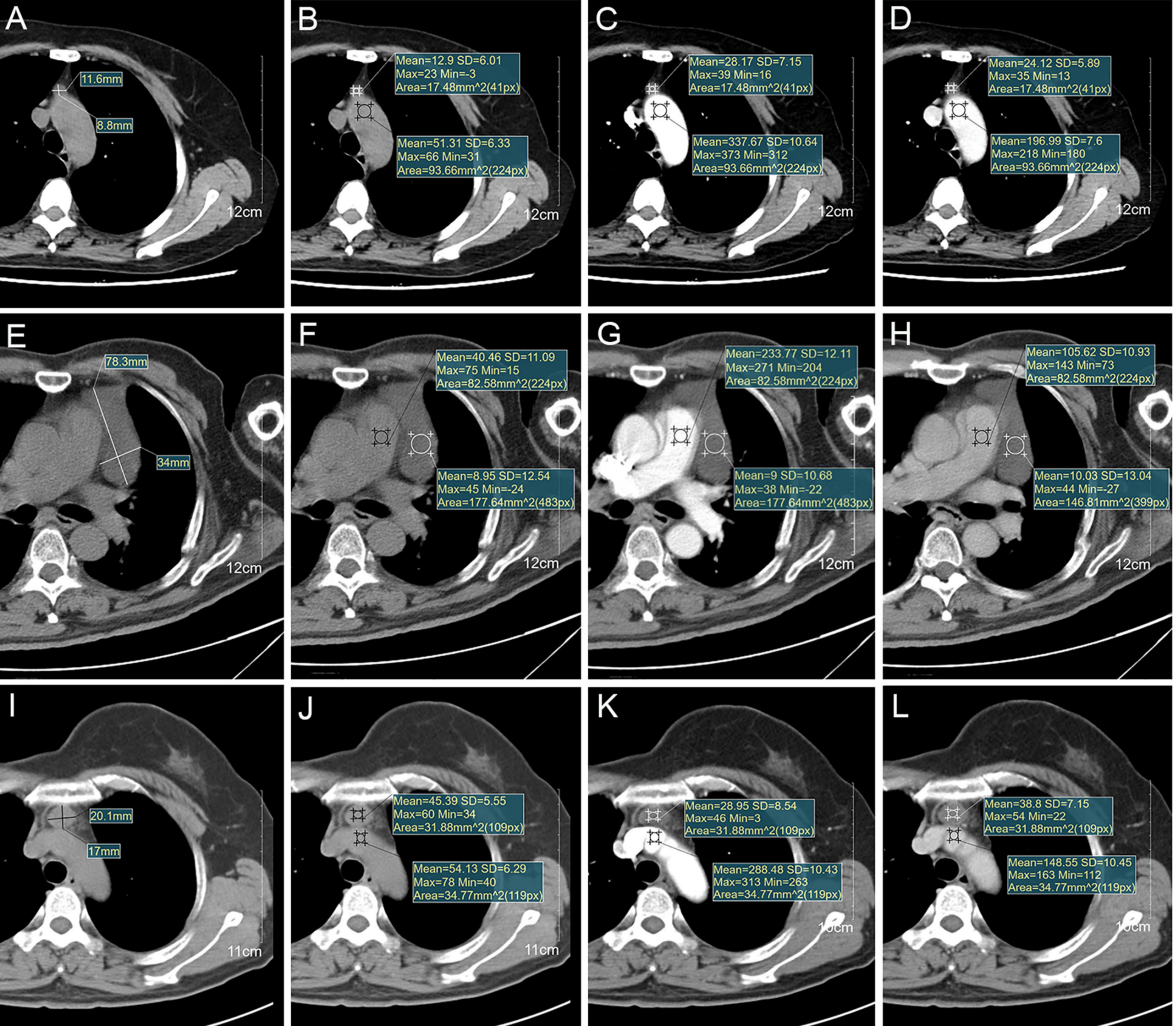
(A-D) Images of a thymic cyst with increased postcontrast attenuation values on CE-CT in 62-year-old woman (age in 2018). Imaging shows (A) a thymic cyst measuring 11.6×8.8 mm, (B) 12.9HU with aortic arch measuring 51.3HU on nonenhanced CT, (C) 28.2HU with aortic arch measuring 337.7HU on arterial phase, and (D) 24.1HU with aortic arch measuring 197.0HU on venous phase of CE-CT. (E-H) Images of a thymic cyst with stable postcontrast attenuation values on CE-CT in 65-year-old man (age in 2017). Imaging shows (E) a thymic cyst measuring 78.3×34 mm, (F) 9.0HU with main pulmonary artery measuring 40.5HU on nonenhanced CT, (G) 9.0HU with main pulmonary artery measuring 233.8HU on arterial phase, and (H) 10.0HU with main pulmonary artery measuring 105.6HU on venous phase of CE-CT. (I-L) Images of a thymic cyst with decreased postcontrast attenuation values on CE-CT in 53-year-old woman (age in 2018). Imaging shows (I) a thymic cyst measuring 20.1×17.0 mm, (J) 45.4HU with brachiocephalic trunk measuring 54.1HU on nonenhanced CT, (K) 29.0HU with brachiocephalic trunk measuring 288.5HU on arterial phase, and (L) 38.8HU with brachiocephalic trunk measuring 148.6HU on venous phase of CE-CT.
